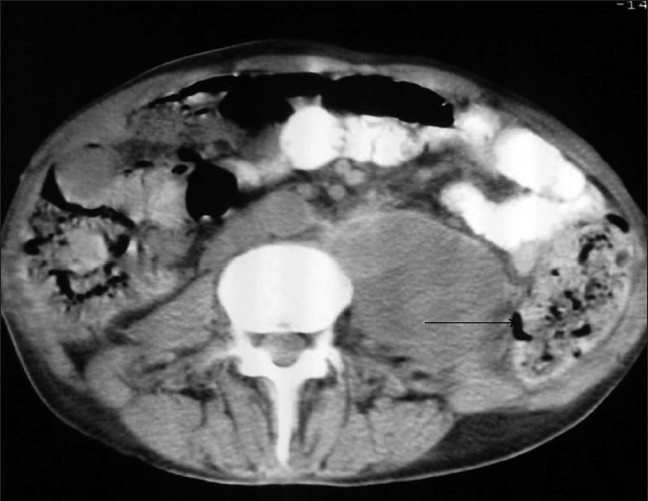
Spiral CT scan shows abscess
